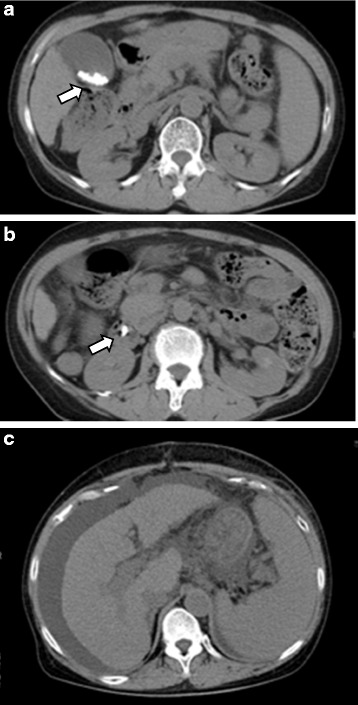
a–c Pretransplant abdominal CT imaging studies in our case 1 before primary operation showed stones in the gallbladder (*arrow* in a) and common bile duct (*arrow* in b). After primary operation, abdominal CT imaging showed shrinking liver and ascites retention (c)
